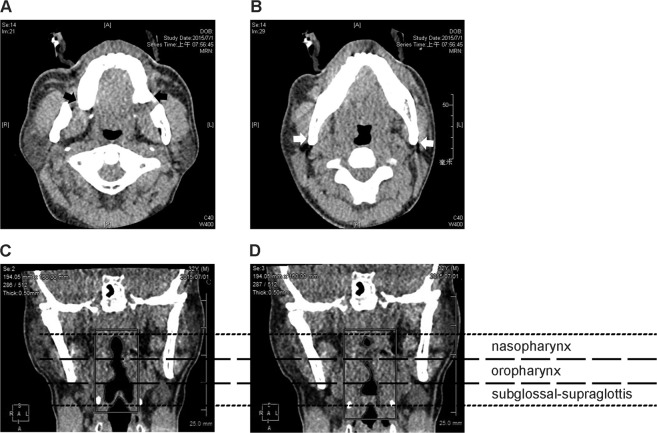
Radiographic landmarks of the parapharynx. The maxillary tuberosities (black arrows) were used as the landmarks of the retropalatal plane (A), and the mandible angles (white arrows) were used as the landmark of the retroglossal plane (B) in axial images. Sites of parapharyngeal lateral wall collapse were rated by comparing the maximal lateral wall length (C) and the minimal lateral wall length (D) of the nasopharynx, oropharynx, and subglosso-supraglottis during an apneic/hypopneic event in coronal images. In this representative patient, complete nasopharyngeal lateral wall collapse, complete oropharyngeal lateral wall collapse, and incomplete subglosso-supraglottic lateral wall collapse were detected.
